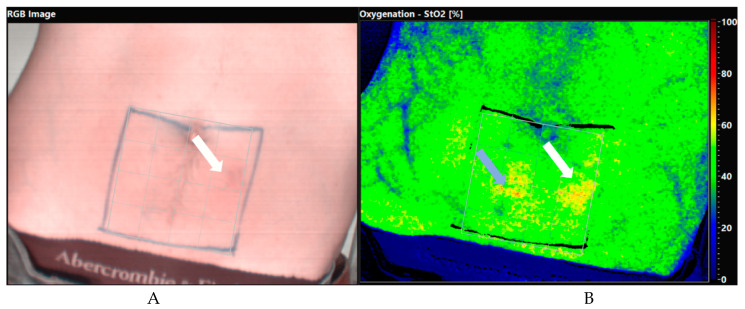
Exemplary depiction of a marked perforator (A) and the corresponding perforator in the HS (Oxygenation StO_2_) image (B). The mark has been removed and is visible palely to not interfere with the HS imaging. The grey arrow shows another possible perforator that had not been referenced with the pencil Doppler; it was not distinctly more prominent than the reference perforator.
